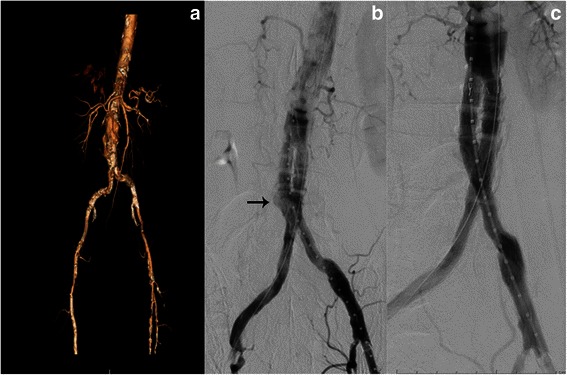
a 3D reconstruction CT preoperatively. b Type 3 endoleak (*arrow*). c Completion angiography
